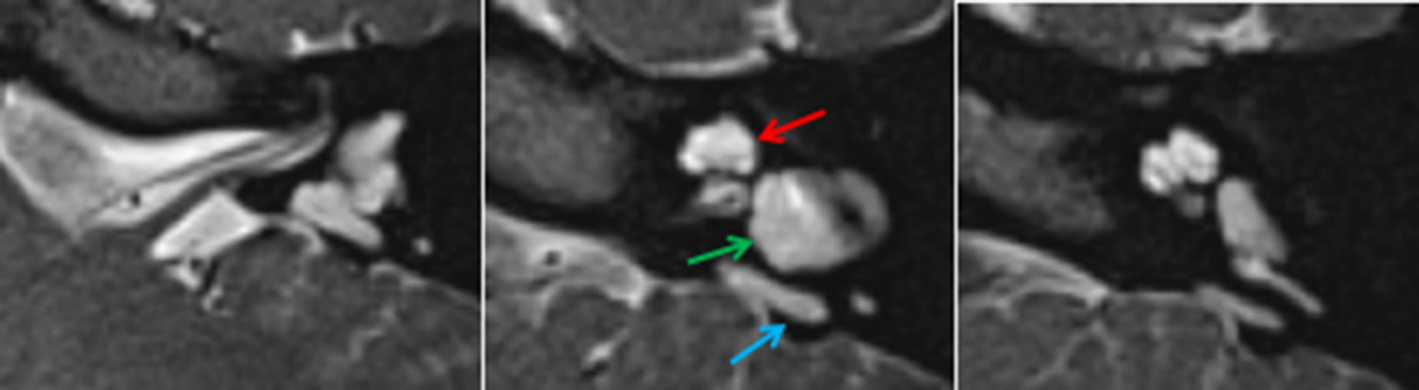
MRI appearances of incomplete partition Type 2, note cystic-like appearance of the upper cochlear turn with flattening of the interscalar septum laterally (red arrow), dilated and dysmorphic vestibule (green arrow) and enlarged endolymphatic sac (blue arrow).
